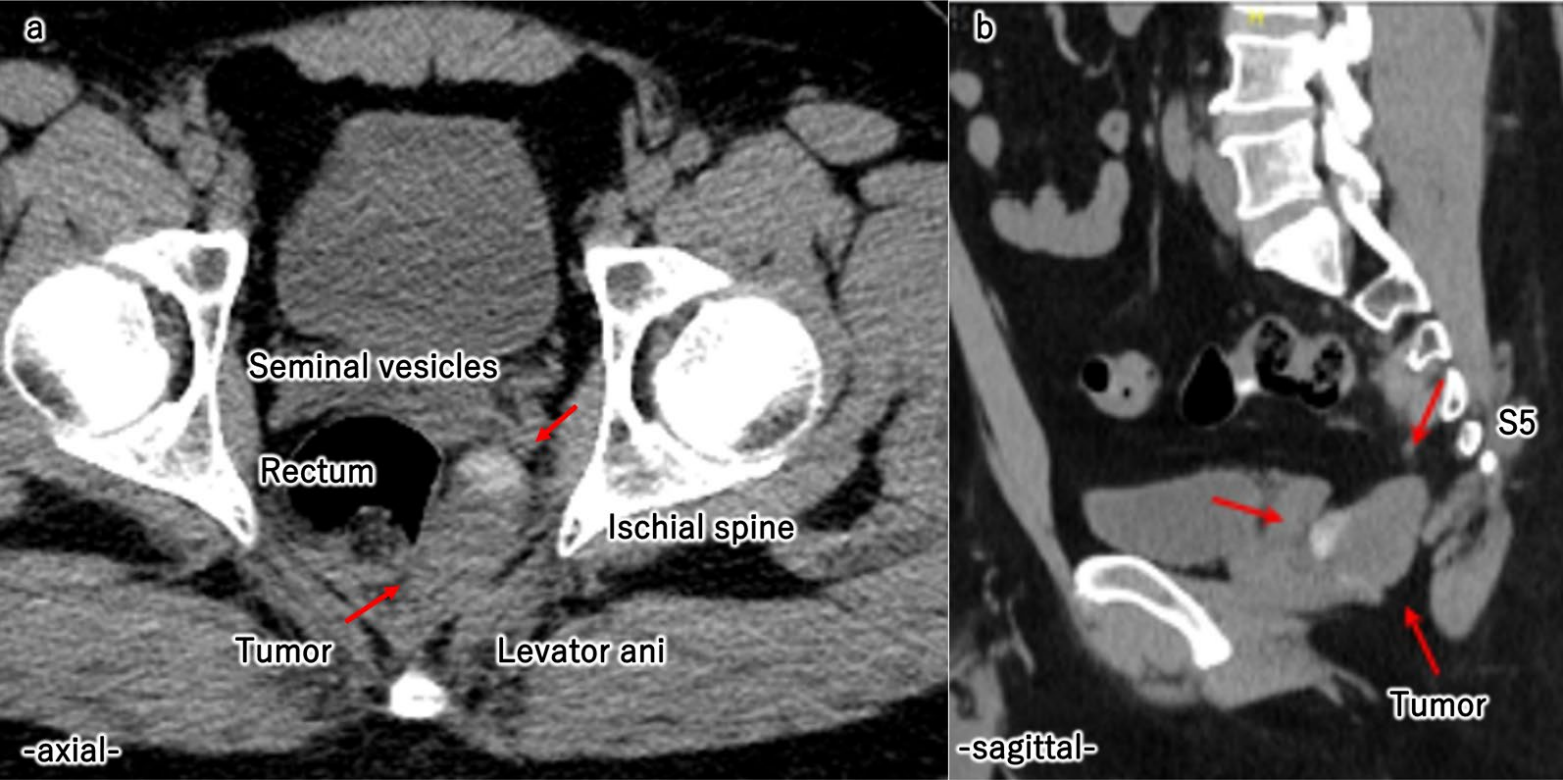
Abdominal contrast-enhanced CT. Contrast-enhanced CT showed a 45 × 40 mm tumor on the left ventral side of the rectum, the right side of the ischial spine, the dorsal side of the seminal vesicles (a), and anterior to the 5th sacrum (b)
